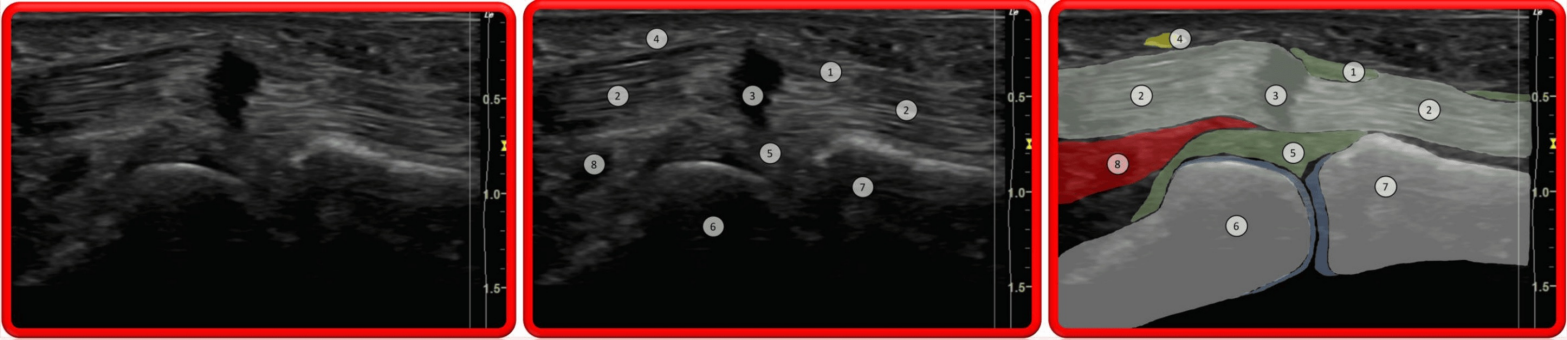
Trigger thumb sonogram Longitudinal view: 1) A1 annular pulley; 2) FPL; 3) FPL anisotropic artifact (frequently observed in cases of triggering with impaired tendon excursion); 4) Thumb radial digital nerve; 5) Palmar plate; 6) Head of first metacarpal; 7) Base of proximal phalanx; 8) FPB. Image taken from the studied patient.
